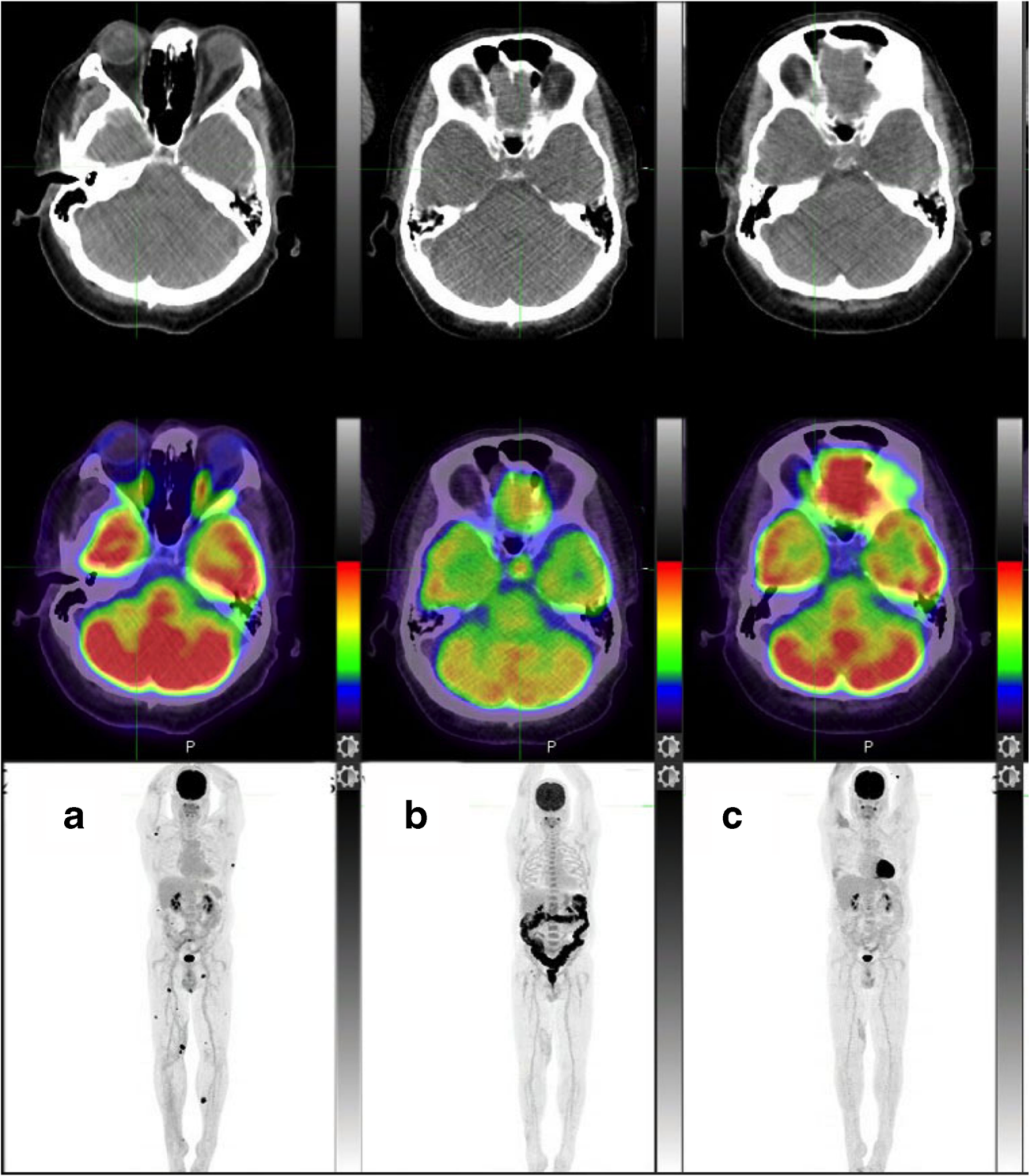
PET/CT imaging in a patient with a previous complete metabolic response of subcutaneous metastases to immunotherapy. a, b Comparison of the baseline maximum intensity projection image (a) with the early posttreatment images (b) shows development of increased uptake in the pituitary fossa on the corresponding fused PET/CT image indicating hypophysitis and diffuse colonic uptake indicating colitis, which were confirmed biochemically and clinically. c Resolution of both complications is apparent after treatment with corticosteroids
